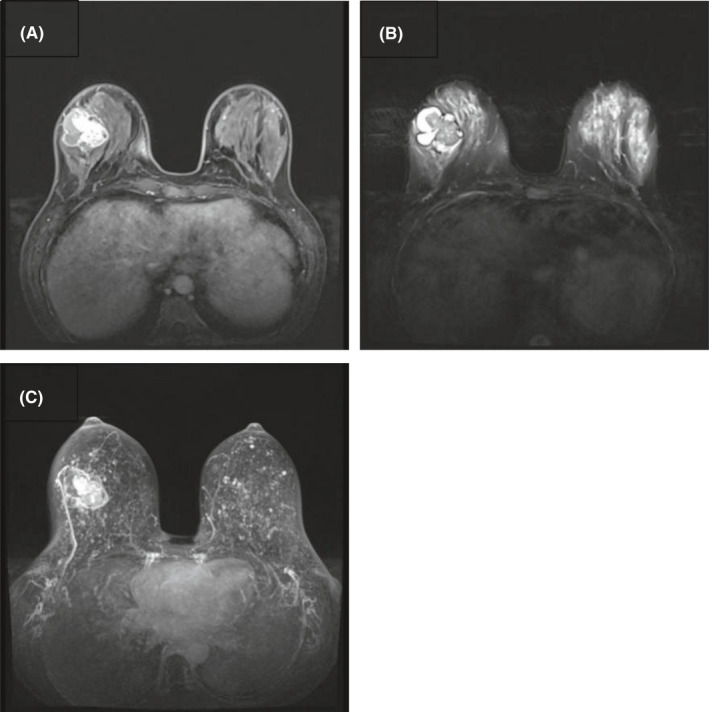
Magnetic resonance imaging of primary breast mucoepidermoid carcinoma. (A) T1‐weighted imaging showed the solid tissue had an equal‐intensity signal with a high‐intensity signal at the periphery of the mass. (B) T2‐weighted imaging showed the solid tissue had a low‐intensity signal with a high signal at the periphery of the mass. (C) Maximum intensity projection showed the lateral thoracic artery of the right breast was thicker than the left breast
